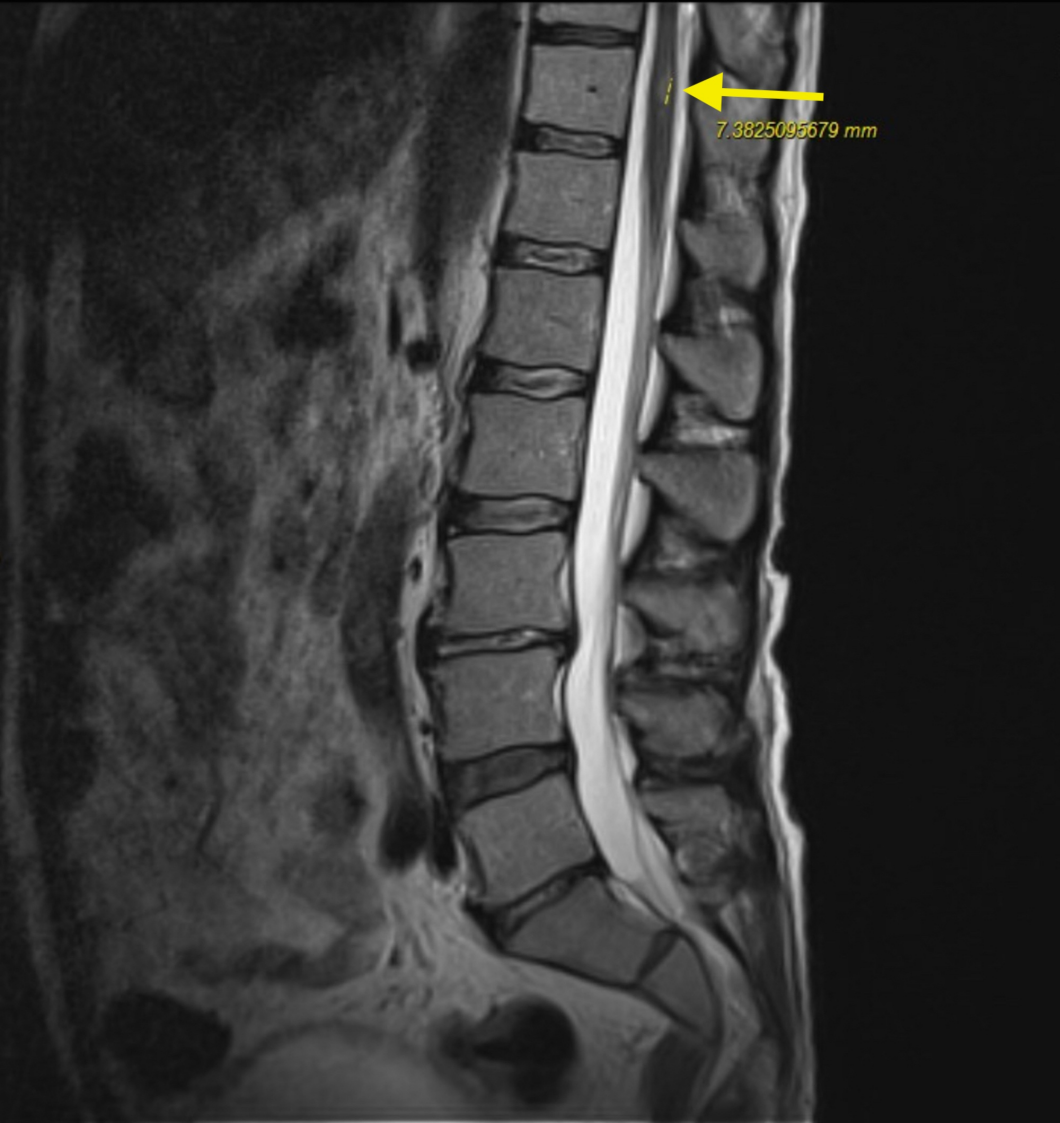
Magnetic resonance imaging (MRI) lumbar spine demonstrating 7 mm lesion and increased signal in superior margin of T11 (yellow arrow).
